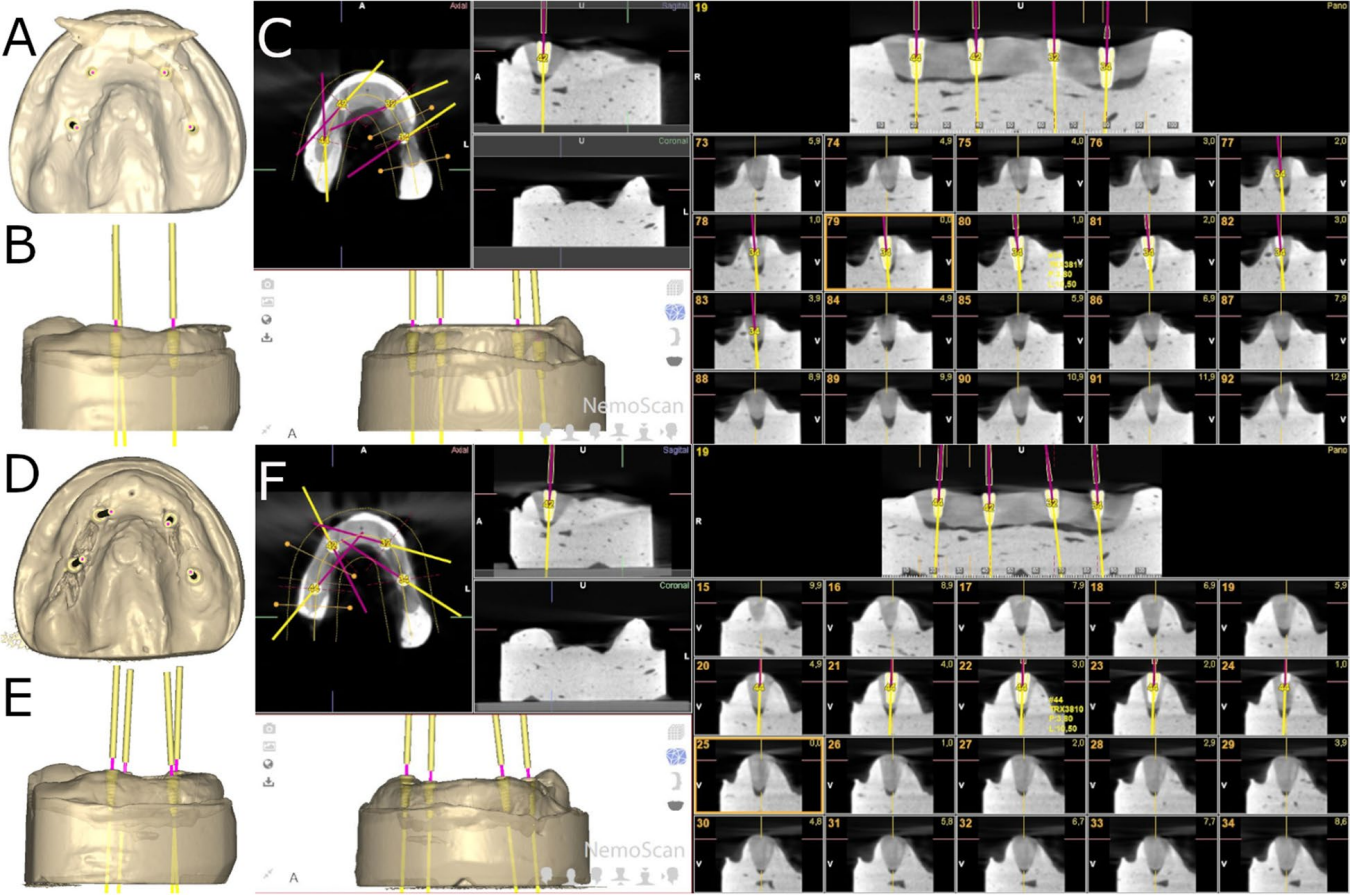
a Coronal, (b) left lateral view and (c) postoperative CBCT scan images of the four dental implant placements with straight parallel pins. d Coronal, (e) left lateral view and (f) postoperative CBCT scan images of the four dental implant placements without straight parallel pins. Yellow and pink lines represent the direction of each dental implant
